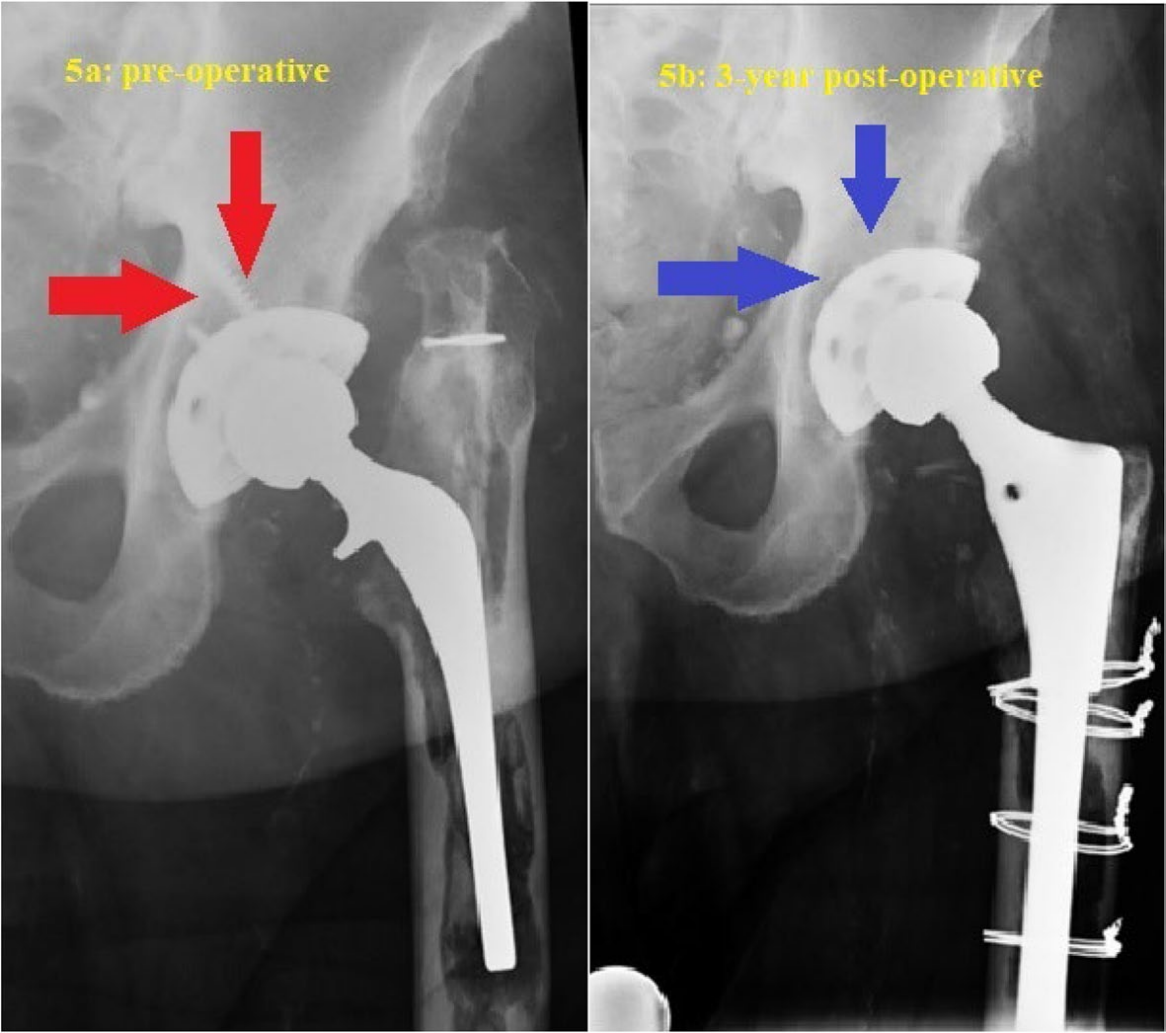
Serial imaging of an 83-year-old male after revision arthroplasty for a loose femoral stem and polyethylene wear. The well-fixed cup was retained, and all screws were removed for stability testing and to allow for retro-acetabular grafting. Demineralized bone matrix was delivered via a syringe and a vinyl urinary catheter. a Pre-operative radiograph showing retro-acetabular osteolysis around the screw (red arrows); b Three-year post-operative radiograph revealing the incorporation of demineralized bone matrix (blue arrows) in the previous osteolytic lesion
